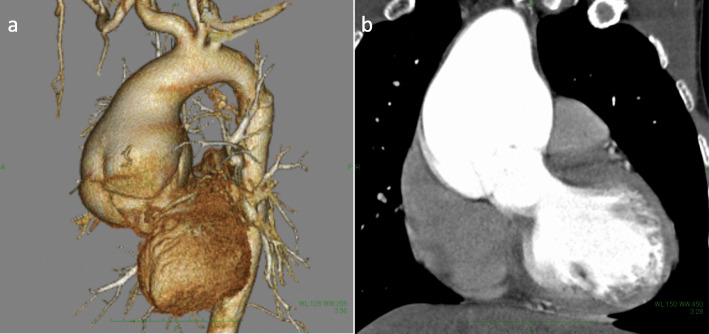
Contrast-enhanced computed tomography scan showing a significantly dilatated aortic root and ascending aorta. Aortic aneurysm was located in the ascending aorta (a), and the maximum diameter of the aortic aneurysm is 88 mm at the sinus of Valsalva (b)
